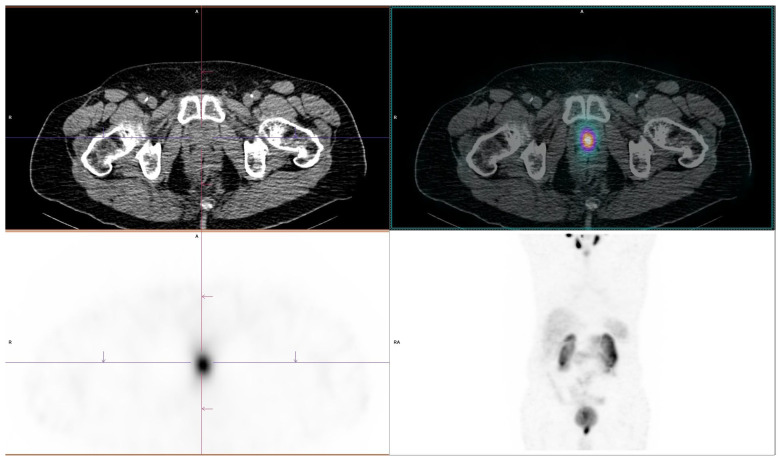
A 75-year-old man with BCR of prostate cancer after radical prostatectomy (ISUP grade 2) underwent Tc-99m-PSMA SPECT/CT at a PSA of 2.95 ng/mL, which demonstrated pathological tracer uptake in the prostate bed, highly suspicious for local recurrence. Multiparametric MRI was performed 2 weeks later, which revealed a focal area of increased T2 signal with restricted diffusion in the prostate bed, also suspicious for recurrent disease. Based on the concordant SPECT/CT and MRI findings, the patient received salvage radiotherapy to the prostate bed, resulting in a subsequent decline in serum PSA.
